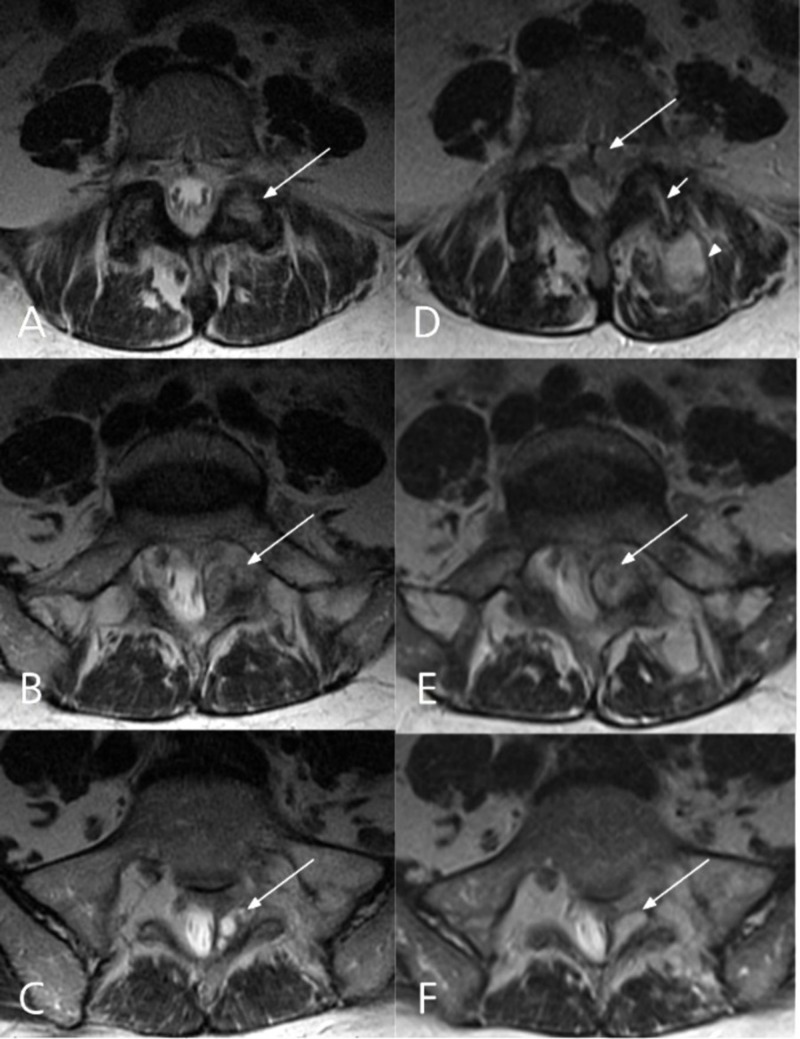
Comparative axial T2-weighted MRI images 2 months (A, C, E) and 15 months (B, D, F) after initial presentation The septic arthritis of the left L5-S1 facet joint has complicated into an epidural abscess (D). A: Right L5-S1 facet involvement with inflammatory change (arrow). B-C: Complex multi-cystic lesion originating from left L5/S1 facet (arrows). D: Isointense posterior and anterolateral epidural collections (long arrow), widening of the left L5-S1 facet joint (short arrow) and paraspinal muscle inflammatory changes (arrow head). E: The cystic lesion has increased in size (arrow). F: The content of the cystic lesion has changed and is now more homogenous (arrow).
